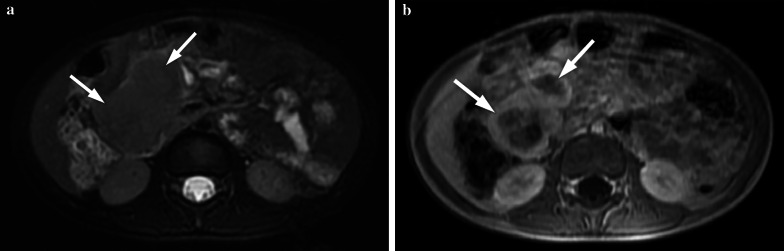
a, b A 3-year-old girl with inflammatory pseudotumor. She had fever and weight loss for the last 3–4 months. In physical examination, a mass was detected in the abdomen. a Axial fat-saturated T2-weighted image shows hypointense tumor in the ileum (arrows). b Post-contrast T1-weighted axial image shows peripherally contrast-enhanced tumor with central necrotic parts (arrows). After biopsy, ALK and SMA positive inflammatory pseudotumor was diagnosed
